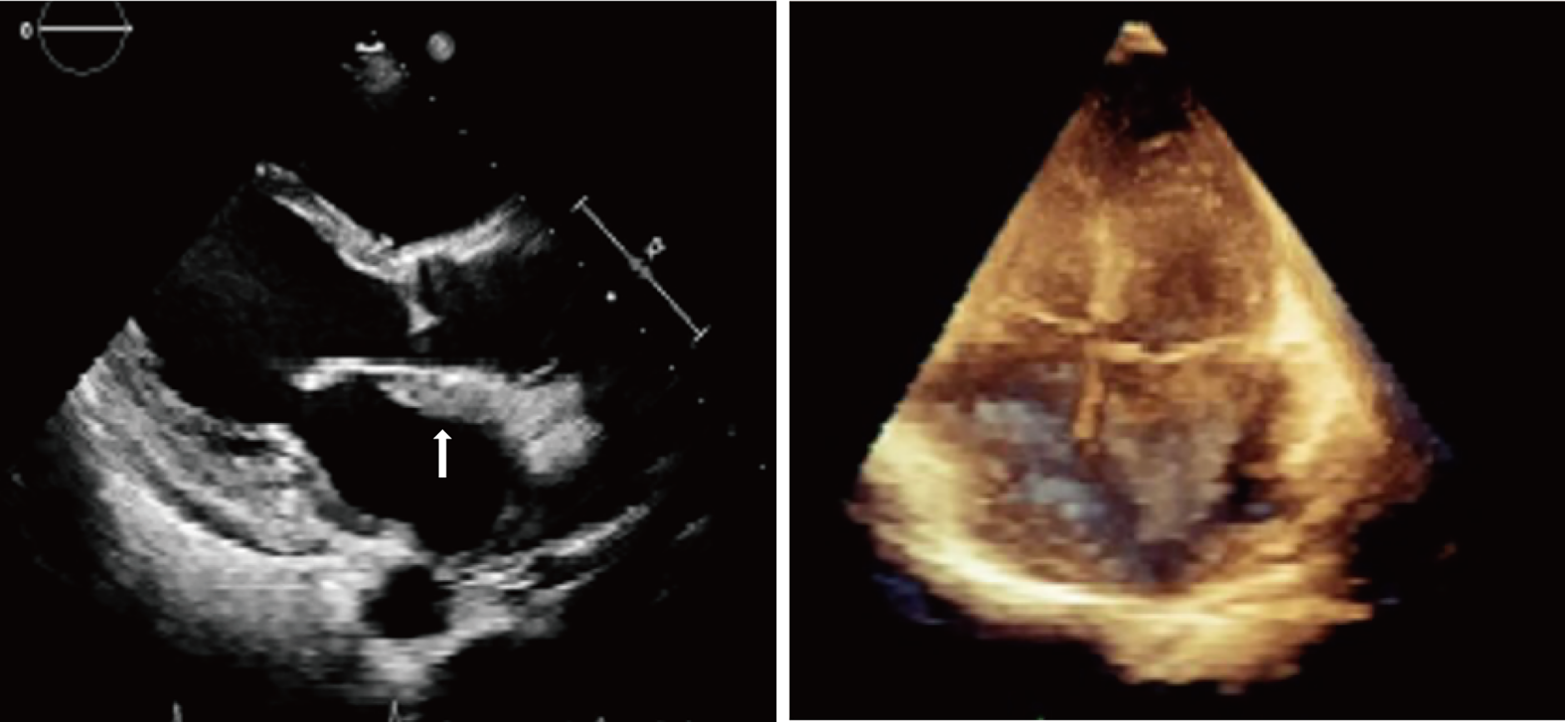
Transthoracic echocardiography showed a homogeneous isoechoic layer thickening of the endocardial surface of the left atrium across the entire left atrial wall.
